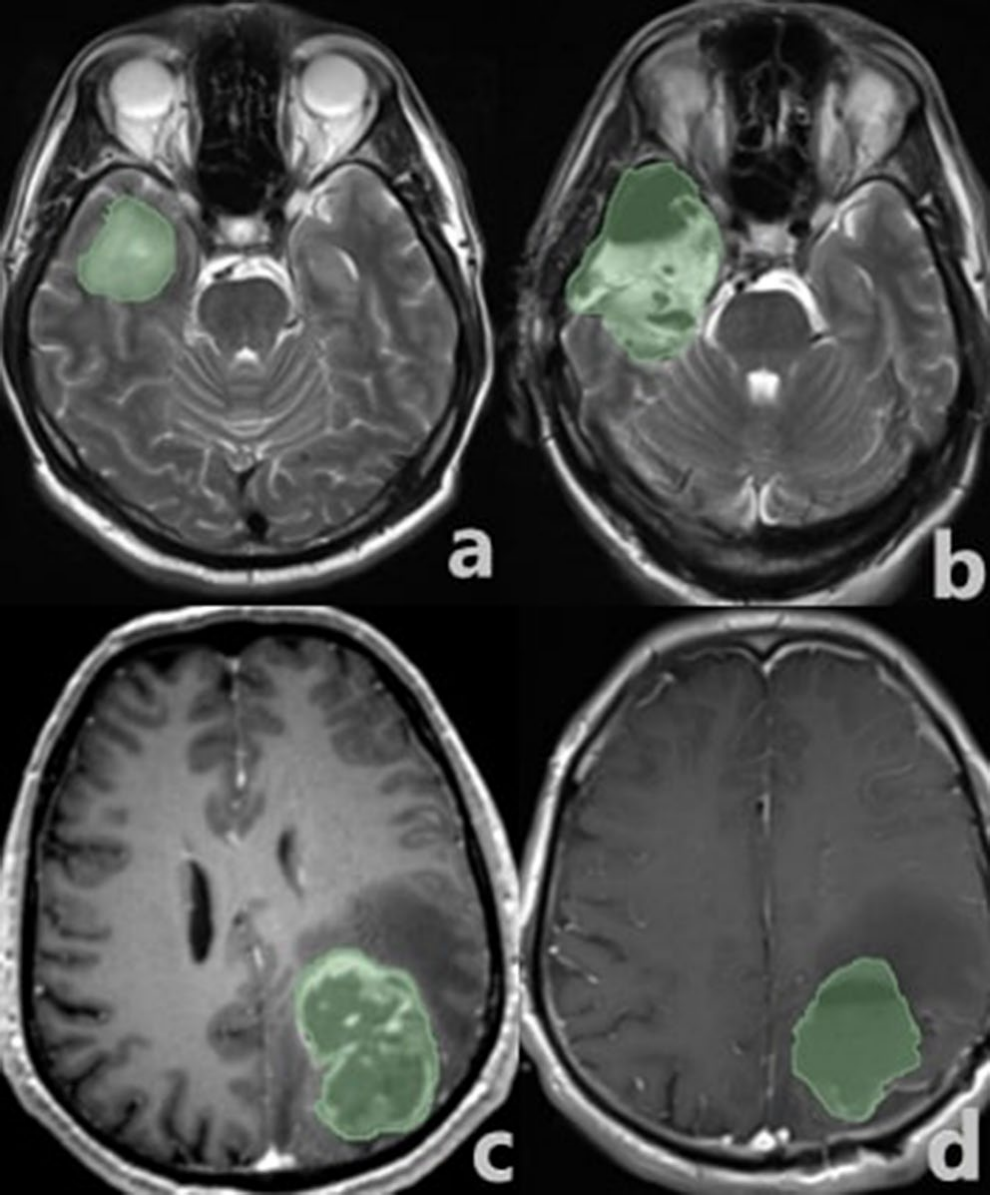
Axial T2W image shows preoperative volumetric analysis of the intraaxial mass located in the right temporal lobe (a); Volumetric analysis of the resection cavity in the intraoperative T2W MRI of the same case is compatible with supratotal resection (b); Preoperative axial postcontrast T1 image (c) shows volumetric analysis of the intraaxial mass in the left parieto-occipital; Volumetric analysis of the resection cavity in the intraoperative axial postcontrast T1W MRI of the same patient is compatible with gross total resection (d)
